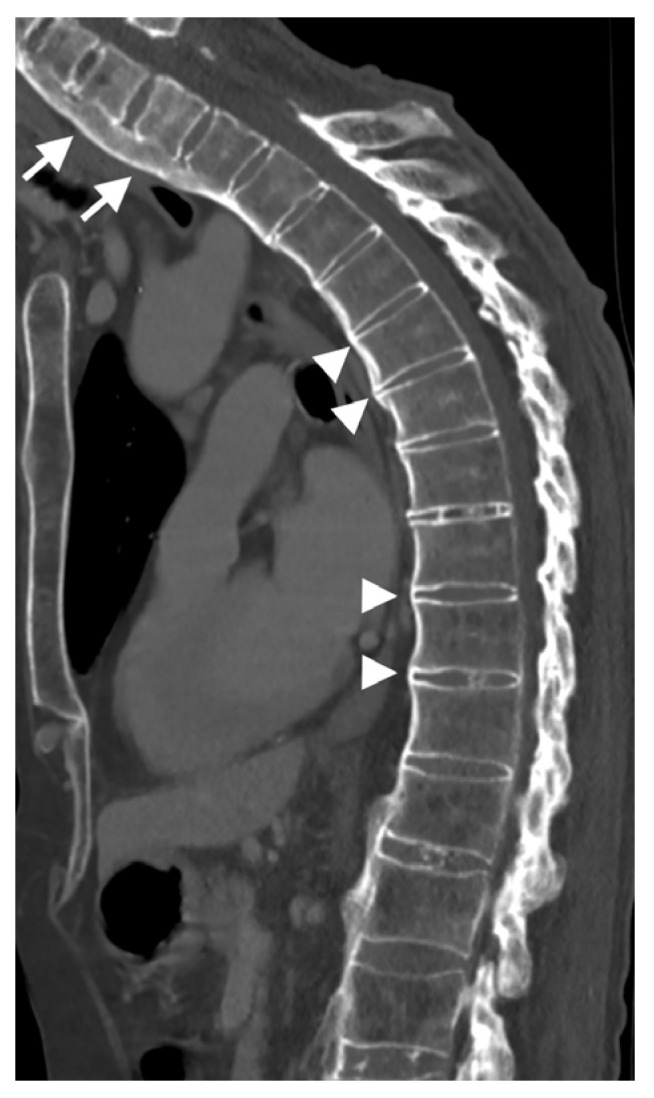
A sagittal CT reconstruction of the cervical and thoracic spine of a 60-year-old patient. There are thick-flowing osteophytes on the cervical spine (arrows), while thin slender vertical syndesmophytes (arrowheads) are in the thoracic spine.
